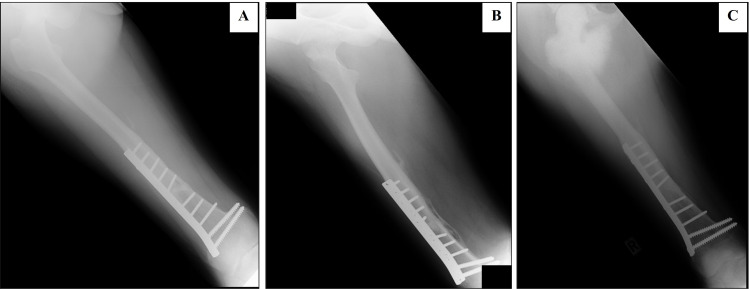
Plain radiographs of a closed fracture of the distal third right femur stabilized with a broad dynamic compression plate The fracture united with thick periosteal reaction and callus formation. No bony lesion was seen (A, B). Three years after the surgery, an osteoblastic lesion involving the metaphysis of the proximal ipsilateral was seen. There were extensive periosteal reaction and extra-osseous extension of the lesion medially. No obvious extension to the distal half of the femur. The femoral plate remained in situ (C).
